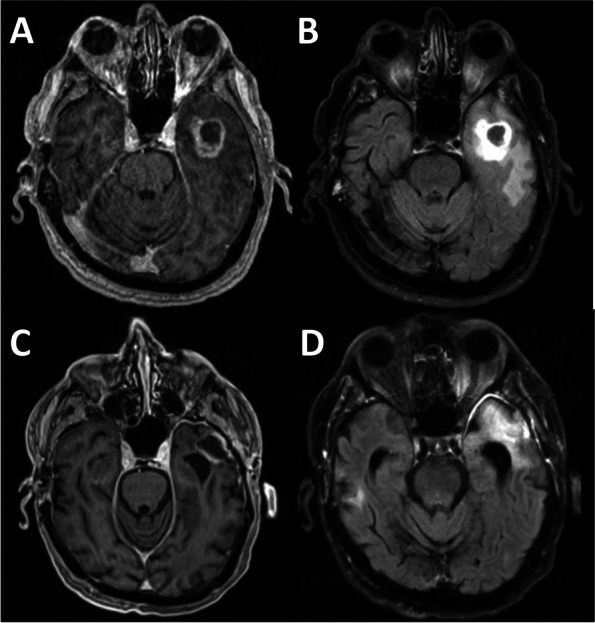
IDH-1 wild-type glioblastoma before and after surgery, radiation, and chemotherapy. MRI from post-gadolinium T1-weighted MP-RAGE (A) and FLAIR (B) sequences demonstrating irregular cystic enhancement and adjacent cerebral edema. The surgical cavity after gross total resection and external beam involved-field radiation as seen on the post-gadolinium T1-weighted MP-RAGE (C) and FLAIR (D) sequences
